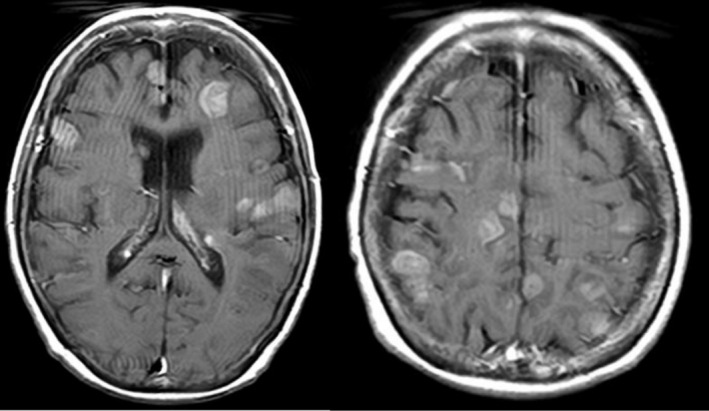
Contrast‐enhanced brain MRI on Day 7 post‐tarlatamab administration. Multiple relatively homogeneous enhancing masses were found in the brain parenchyma, consistent with multiple brain metastases.
